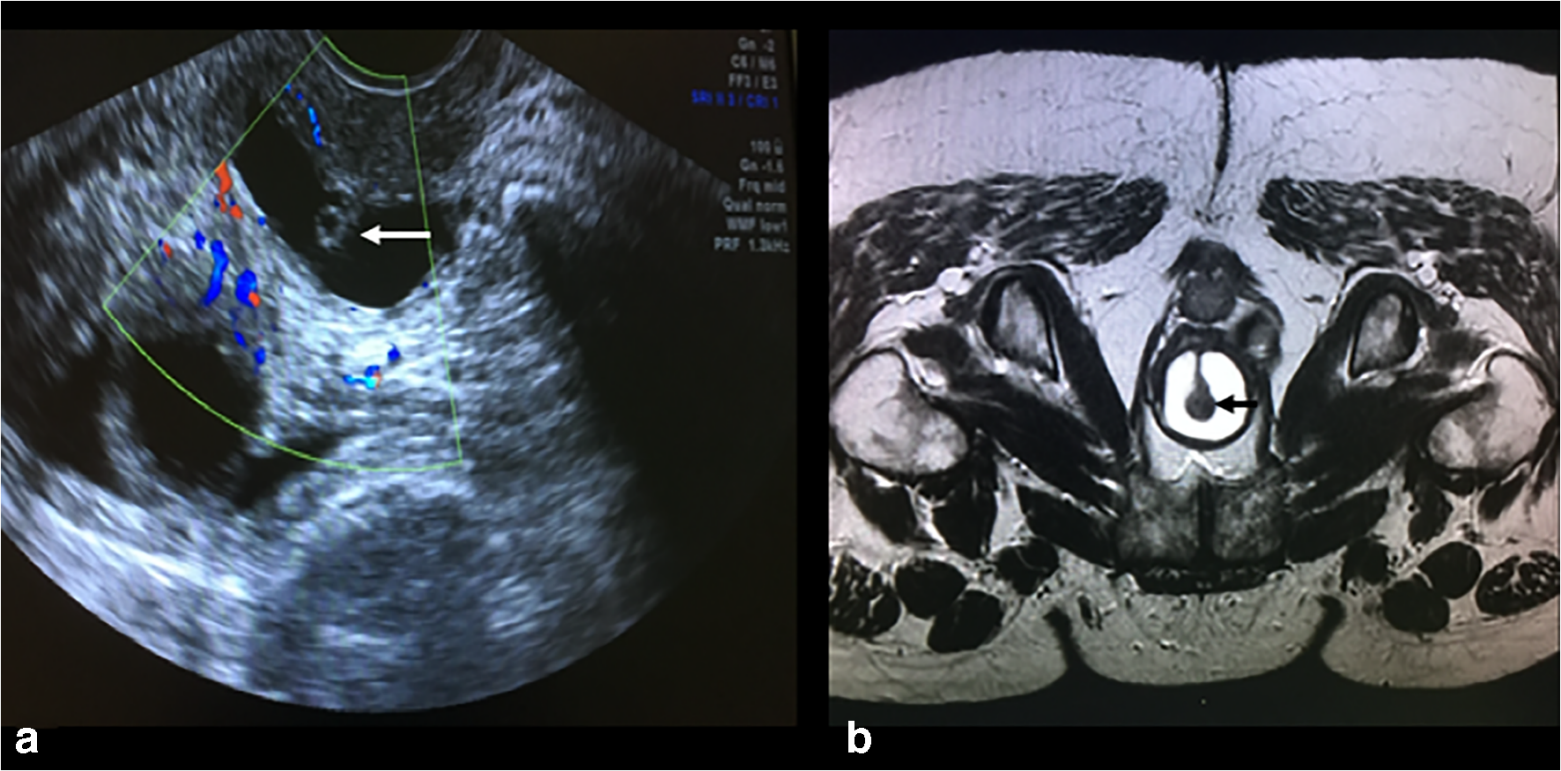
a Transvaginal ultrasound showing the urethra surrounded by the diverticulum. b MRI showing the same part of the urethra. The* arrows* demonstrate the urethra suspended within the diverticulum
